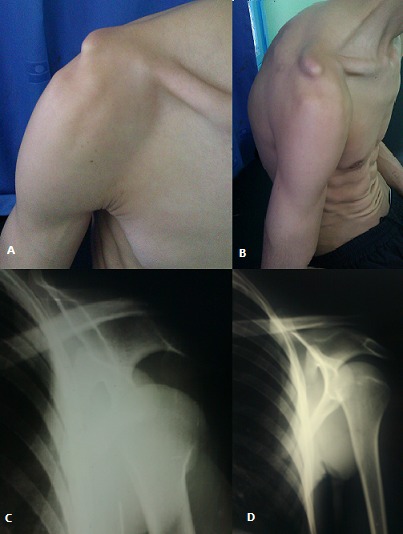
A) aspect clinique de la disjonction acromio claviculaire de face; B) aspect clinique de la disjonction acromio claviculaire de profil; D)radiographie de l'épaule de face avant réduction montrant l'association luxation gléno humérale et disjonction acromio claviculaire; E) radiographie post réduction de la luxation de l'épaule montrant la luxation acromio claviculaire
